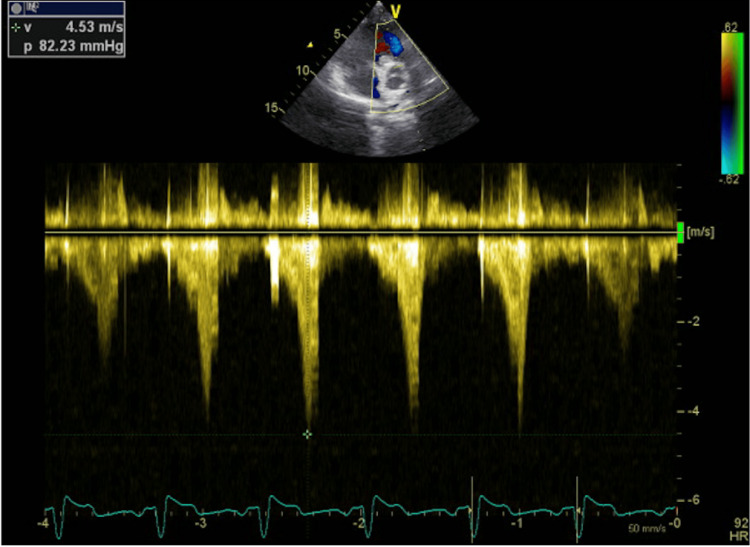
Continuous-Wave Doppler Echocardiography Demonstrating Severe Pulmonary Valve Stenosis. A markedly increased transvalvular flow velocity is seen, with a peak velocity of 4.53 m/s and a maximum systolic gradient of 82.23 mmHg, consistent with severe pulmonary valve stenosis. The Doppler profile reflects significant right ventricular outflow tract obstruction.
